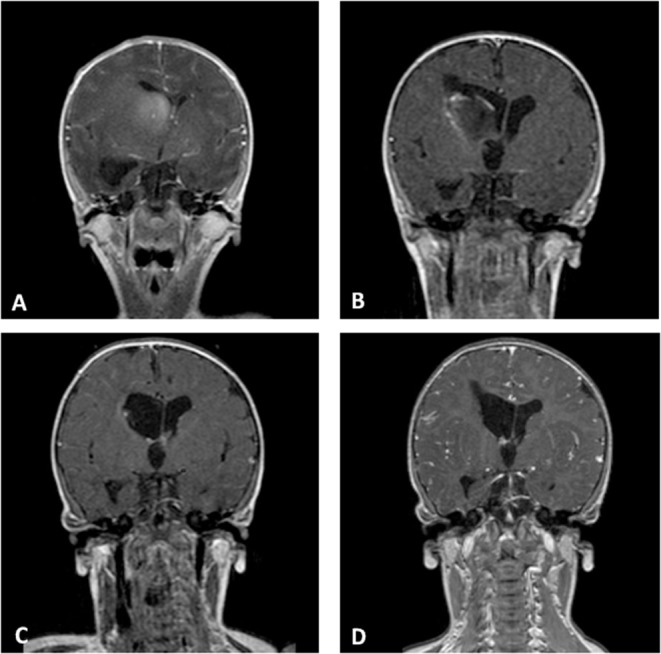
Coronal Gd-enhanced T1-weighted MR scans of a CNS embryonal tumor NOS. (A) Preoperative images demonstrating the intraventricular tumor at the right caudate nucleus. (B) Postoperative scans after septostomy and biopsy of the lesion. (C) Complete response after high-dose chemotherapy and ASCT. (D) Last MR follow-up.
